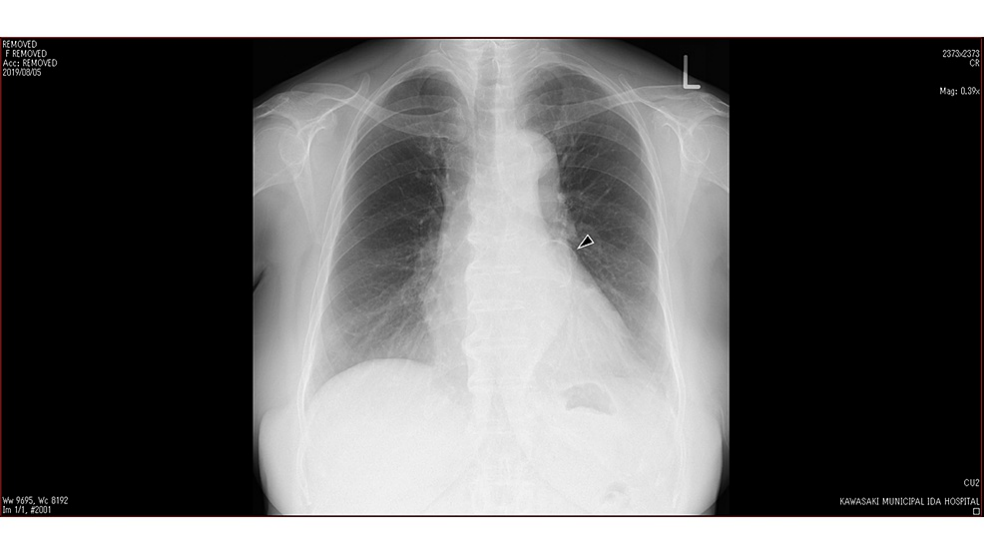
Chest X-ray Chest X-ray shows a round, hypoechoic lesion (black arrow) with calcification.
